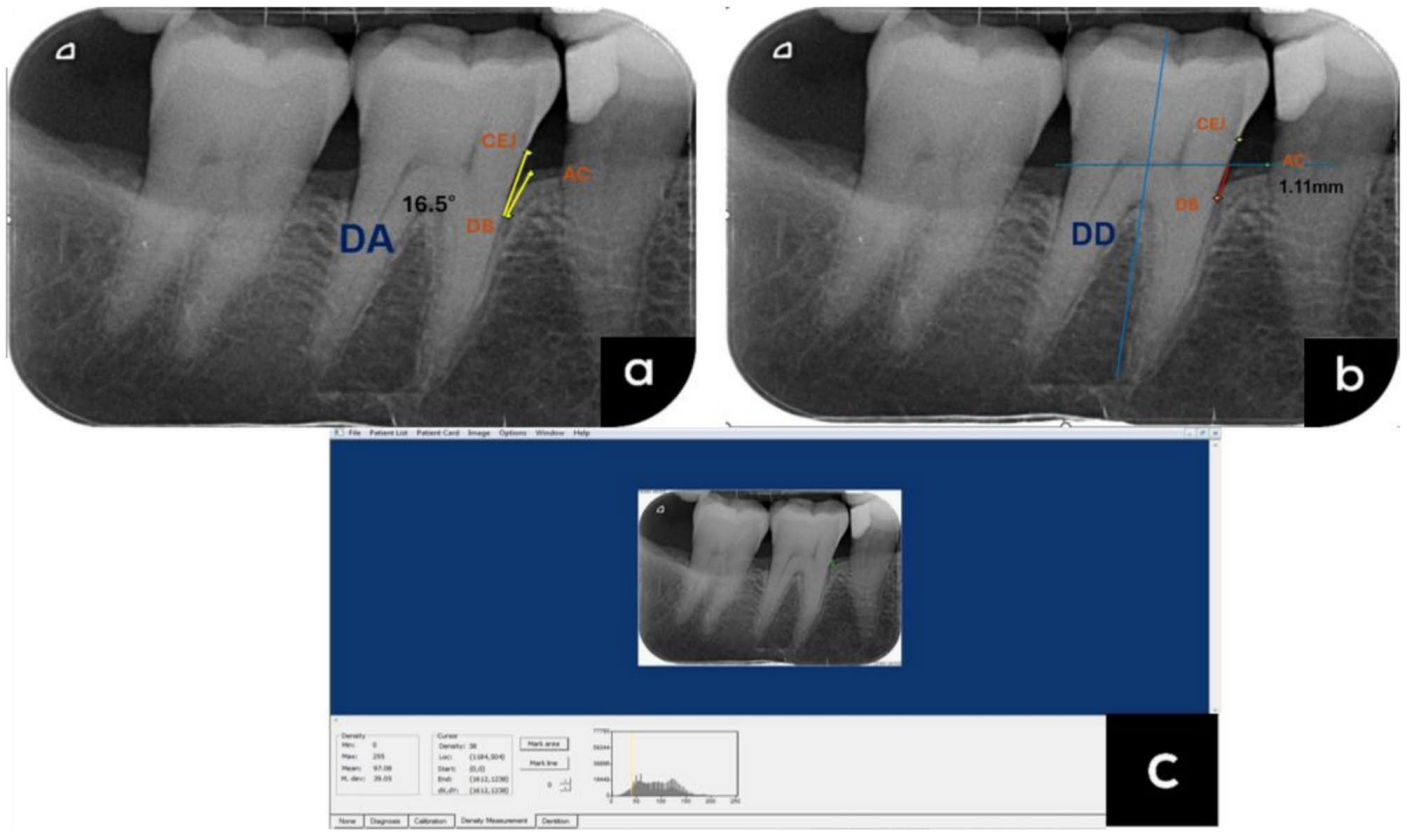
Digitized radiographic images (a) showing bone angle baseline measurements using ImageJ software (b) bone defects and the recorded linear measurements using ImageJ software (c) bone defect density measurement using Digora software
